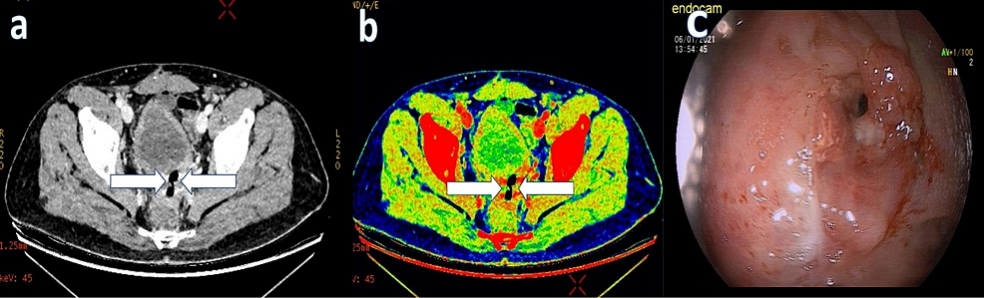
A 43-year-old male patient, who was operated on for rectal cancer, showed loss of integrity in the rectosigmoid anastomosis line and free air in its neighborhood in the IV contrast-enhanced abdominopelvic CT performed with DECT. It was considered a closed perforation. Transmural loss of wall enhancement on (a) 45 keV virtual monoenergetic images (white arrow) and (b) iodine overlay map images (white arrow). (c) Late anastomotic leak in the rectosigmoid anastomosis line.
